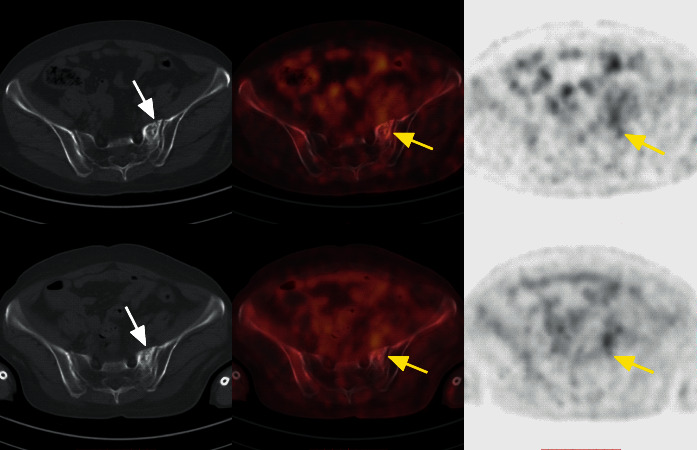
A 56-year-old woman who underwent pelvic radiotherapy for cervical cancer developed hip pain at 9 months after radiotherapy. Focal FDG uptake (SUVmax, 2.6) in the left sacral ala (yellow arrow) was found in the axial PET image, while the low-dose CT showed high density and a very small right anterior sacral fracture line (white arrow). Follow-up FDG PET/CT at 6 months after diagnosis of SIF. The bone density increased, and the metabolic intensity decreased.
